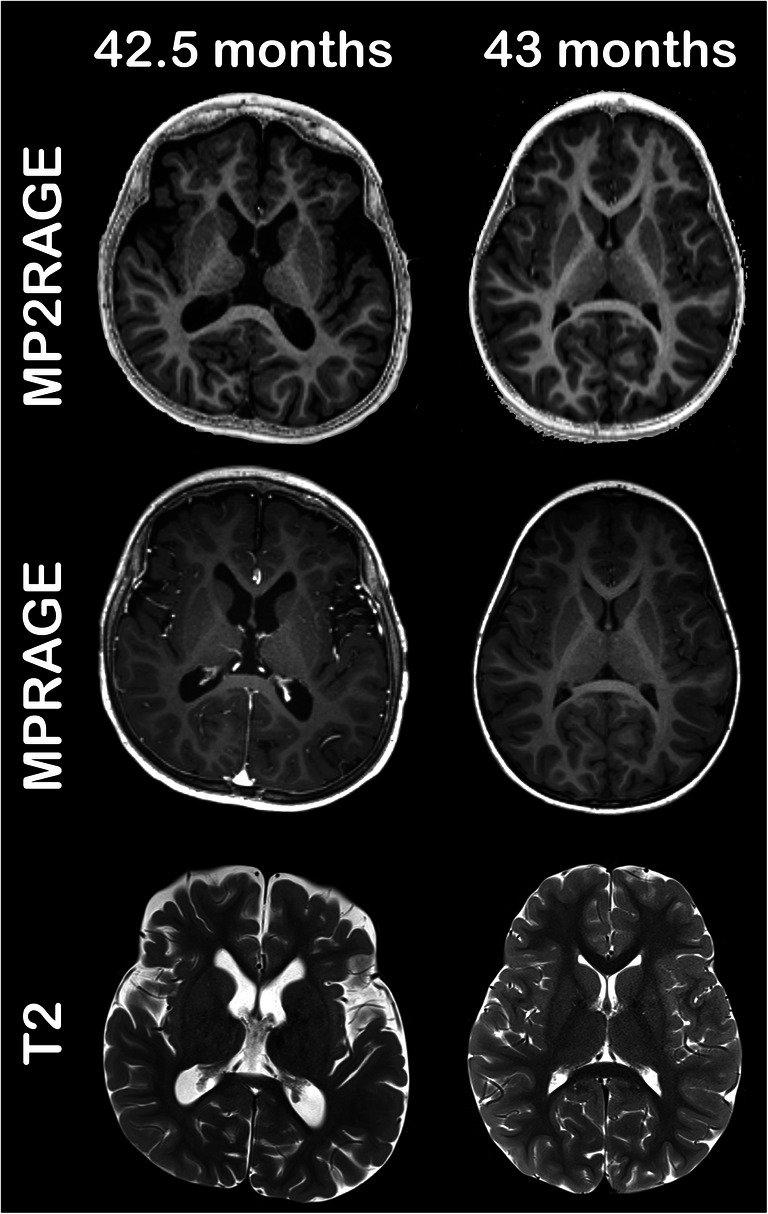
A 42.5-month-old boy with brain atrophy and unspecified epilepsy. Axial MP2RAGE (magnetization-prepared 2 rapid acquisition gradient echoes), contrast-enhanced MP-RAGE (magnetization-prepared rapid acquisition gradient echo) and T2-weighted images show very similar signal intensities in the posterior limb of the internal capsule as in an MRI-negative peer (a 43-month-old girl; her MP-RAGE image is not contrast enhanced). Note the different relaxation rate (R1) values in the posterior limb of the internal capsule in Fig. 3, which cannot be appreciated qualitatively on conventional sequences (right: 0.99±0.05 s^−1^, left: 1.00±0.05 s^−1^ for the boy; right: 1.08±0.04 s^−1^, left: 1.07±0.04 s^−1^ for the MRI-negative girl)
